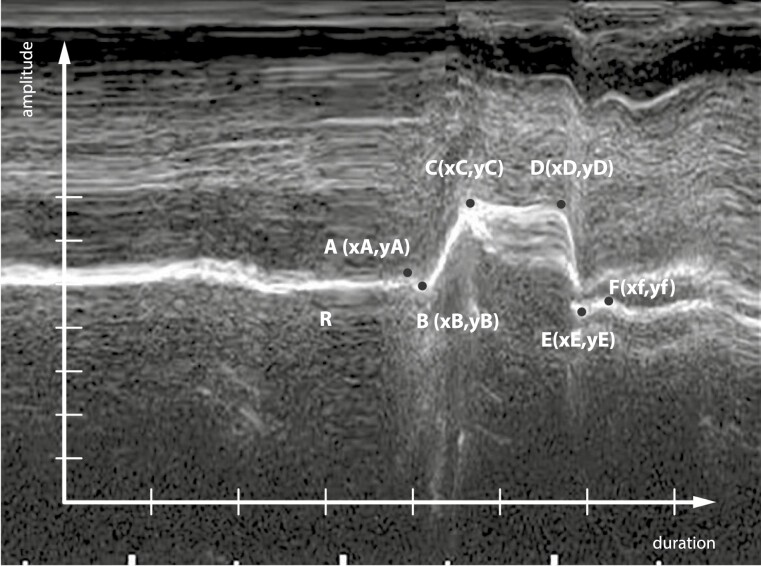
M-mode tongue ultrasonogram with the ultrasound probe’s centre line set in the middle of the tongue and presentation of swallowing phases (I, IIa, IIb, IIIa, and IIIb [15];). In the rest phase (R), the tongue tip is usually positioned on the lingual surfaces of incisors or in contact with the incisive papilla. The swallowing act starts with the shovel phase (A–B; phase I), in which the tongue tip moves cranially, and the middle third of the tongue becomes concave, reflected in the down-movement of the curve in the M-mode ultrasonogram. In the early transport phase (B–C; phase IIa), the tongue moves cranially and distally, the middle third of the tongue approaches the hard palate, and therefore the concavity disappears. The late transport phase (C–D, phase IIb) is characterised by minimal vertical movement of the tongue because of the distal transportation of saliva. In the early final phase (D–E, phase IIIa), the curve in the M-mode ultrasonogram drops because of the lowering of the mouth floor. In the late final phase (E–F; phase IIIb), the tongue returns to the rest position, reflected as a rise in the M-mode curve [15].
